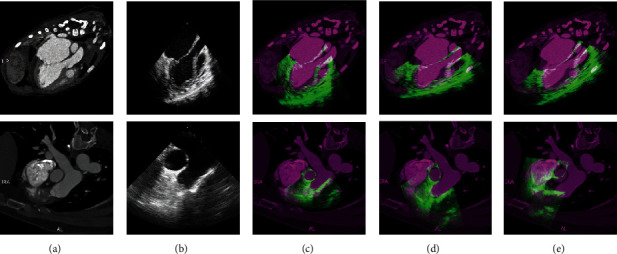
The registration result. (a) Original CT image. (b) Original TEE image. Results of (c) the proposed method. (d) FLIRT. (e) Demons registration.
